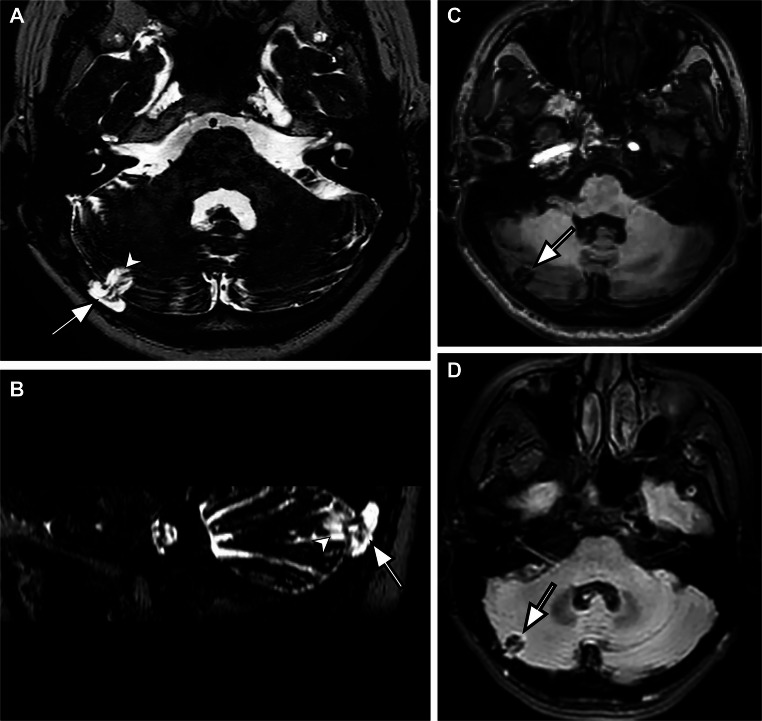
Sixty-five-year-old female patient with vertigo underwent temporal and brain MRI. A Axial and (B) sagittal reformatted 3D-DRIVE images show cerebellar herniation into giant arachnoid granulation (arrow) and encephalomalacia in adjacent parenchyma (arrowhead). C Axial T1-weighted MR image and (D) axial FLAIR image demonstrate parenchymal encephalomalacia and gliosis in both herniated and adjacent parenchyma
